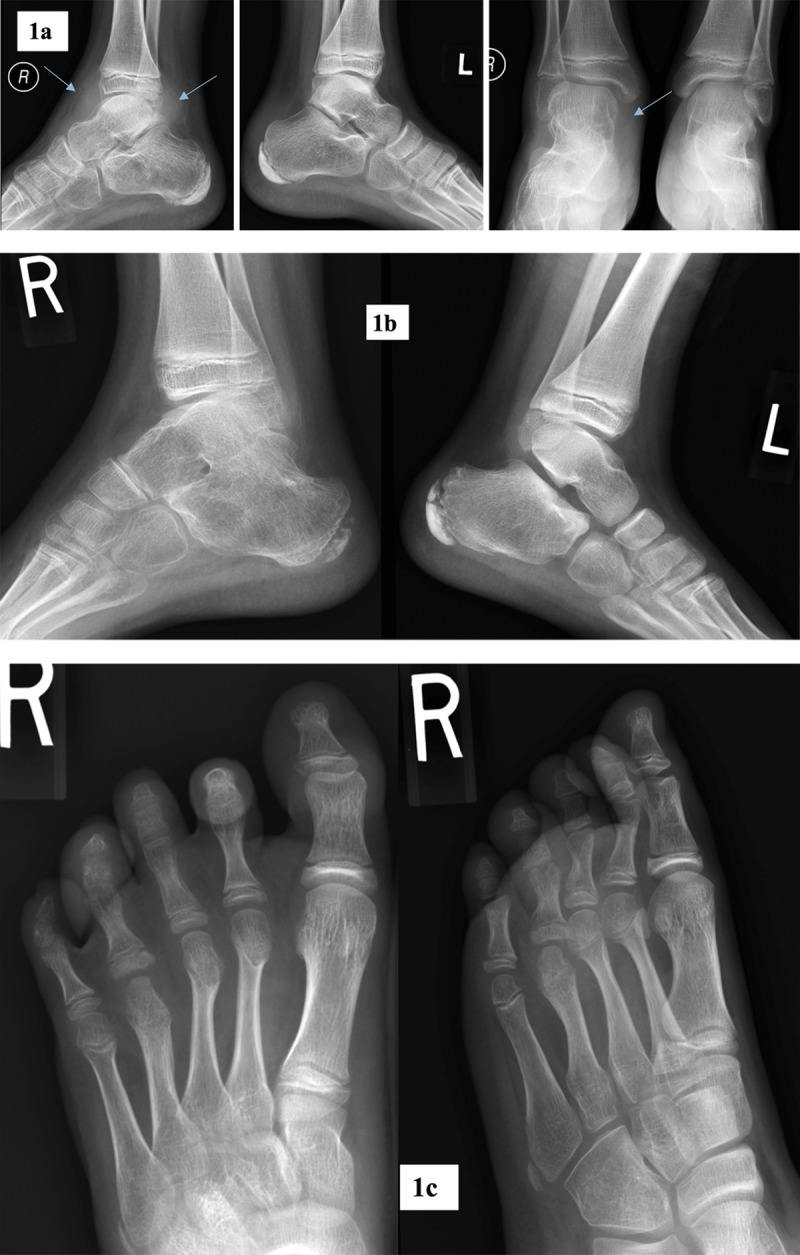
Radiographic abnormalities of foot and ankle. a. Antero-posterior and lateral radiographs of both ankle joints in a 12-year-old male patient with JIA. Marked soft tissue swelling at the level of right ankle joint (arrows). b. Lateral radiographs of ankle joints in a 9-years-old male with JIA. Marked osteoporosis of right talar and calcaneal bones with ankylosis in subtalar joint. Talonavicular joint space narrowing with subchondral sclerosis. Normal left ankle joint. c. Antero-posterior and oblique radiographs of the right foot in a 9-year-old female with JIA. Erosions and subchondral cysts in fourth MTP and PIP joints, periarticular osteoporosis, soft tissue swelling at the level of MTP2 joint.
